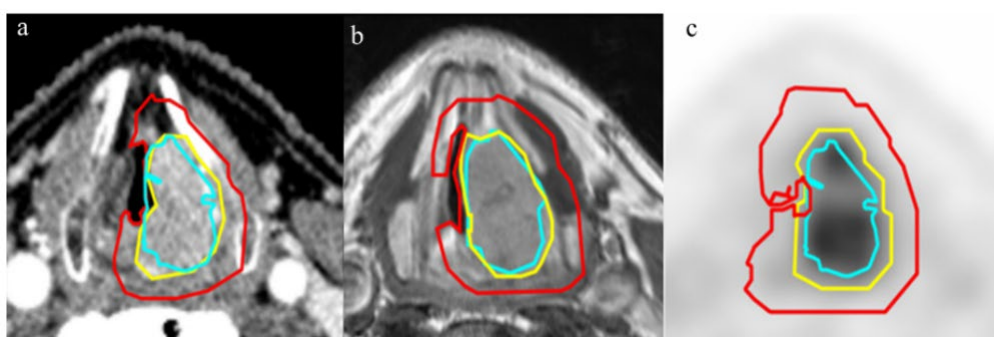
Laryngeal tumor delineated on (a) contrast-enhanced CT, (b) MRI and (c) PET CT [8]
